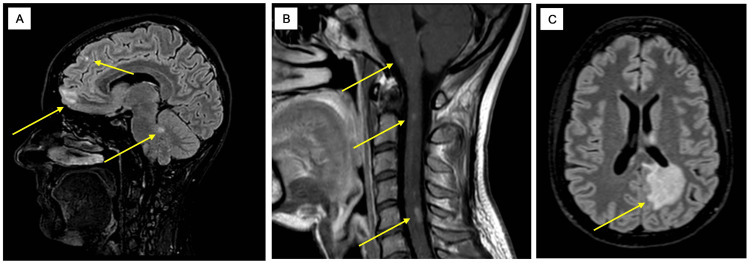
MRI cranium and whole spine, native and with contrast agent. (A) 31.10.2023 T2 FLAIR axial: multiple demyelinating lesions located in the cortex, left frontobasal region, left frontal region, and cerebellum (yellow arrows). (B) 31.10.2023 T1 with contrast agent sagittal: multiple contrast-enhancing demyelinating lesions located in the spinal cord (yellow arrows). (C) 20.12.2023 T2 FLAIR axial: new FLAIR hyperintense confluent demyelinating lesion predominantly located periventricularly in the left parietal region (yellow arrow). FLAIR: fluid-attenuated inversion recovery, MRI: magnetic resonance imaging
